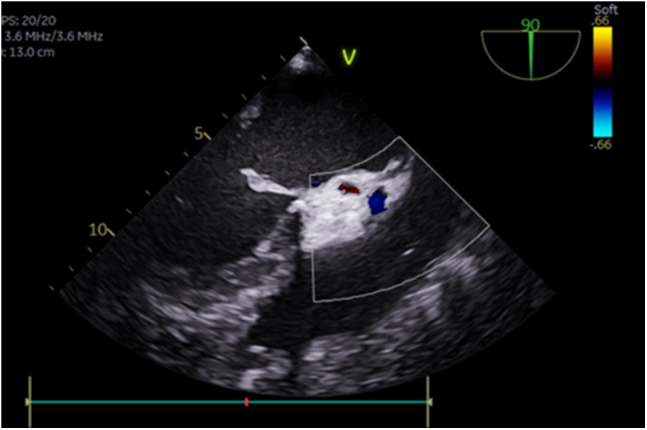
Echocardiogram showing anomalous origin of the right coronary artery.
